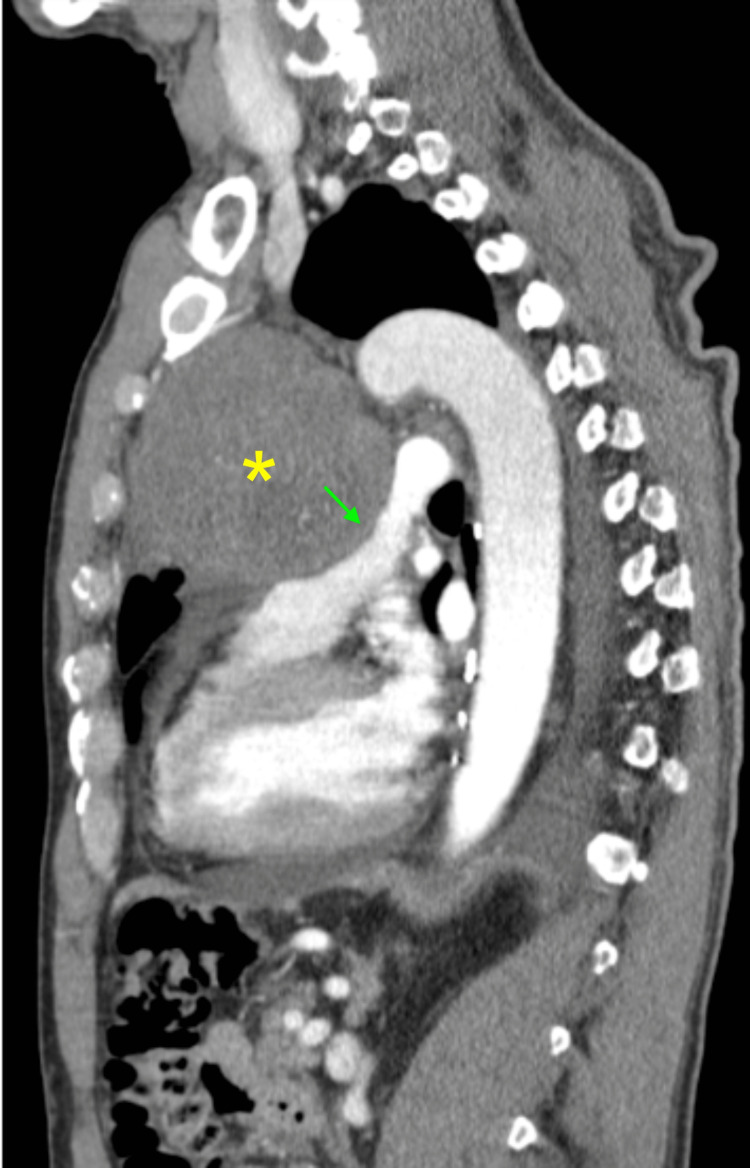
Chest CT, sagittal view The yellow asterisk (*) indicates a large anterior mediastinal mass. The green arrow indicates a mass effect on adjacent cardiopulmonary structures.
